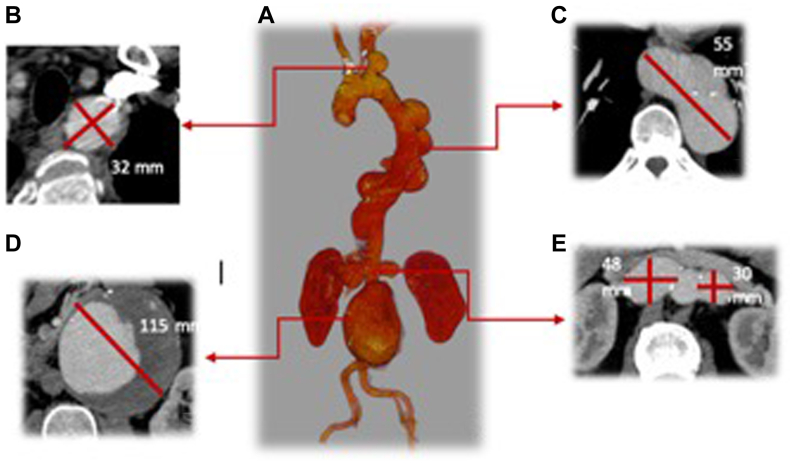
Preoperative computed tomography angiography three-dimensional volume rendering reconstruction (A), showing a left subclavian artery aneurysm (maximum diameter of 32 mm) (B), multiple descending thoracic aorta aneurysms (maximum diameter of 55 mm) (C), a juxtarenal aortic aneurysm with a maximum diameter of 115 mm (D), bilateral renal artery aneurysms (left renal artery maximum diameter of 48 mm, right renal artery maximum diameter of 30 mm) (E).
